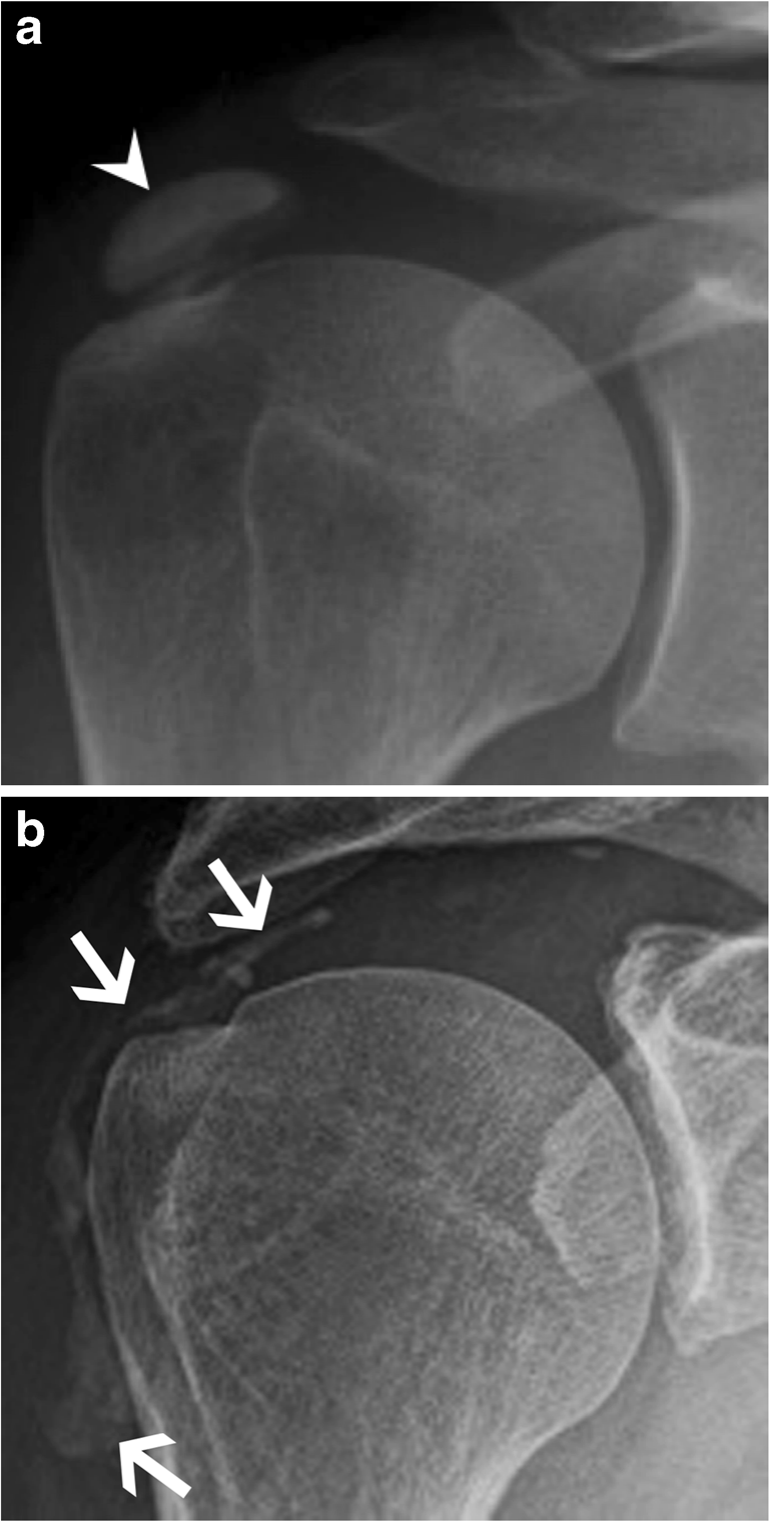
HADD. (a) Anteroposterior radiograph of the right shoulder shows a calcific tendinopathy of the supraspinatus tendon (arrowhead) during formative or resting phase. (b) Anteroposterior radiograph of the right shoulder in a different patient shows migration of the calcifications to the subdeltoid-subacromial bursa (arrows) during the resorptive phase
